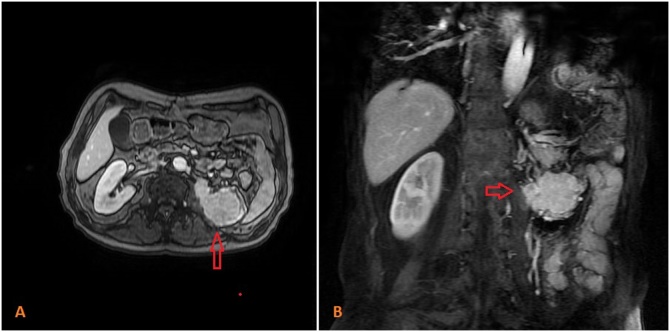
A colonic tumor in abdominal MRI (A and B).
